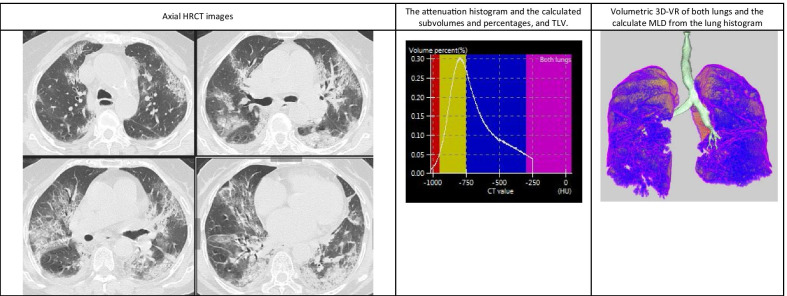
Quantitative CT analysis of COVID-19 pneumonia in 64-year-old man suffering from severe symptoms that required ICU admission with high-flow oxygenation using nasal cannula. Axial HRCT images show multiple large sub-pleural GGOs affecting all lobes of both lungs with few consolidations. Volumetric 3D-VR shows extensive bilateral blue and pink areas representing GGOs and consolidation. The patient had 54.3% disease burden
